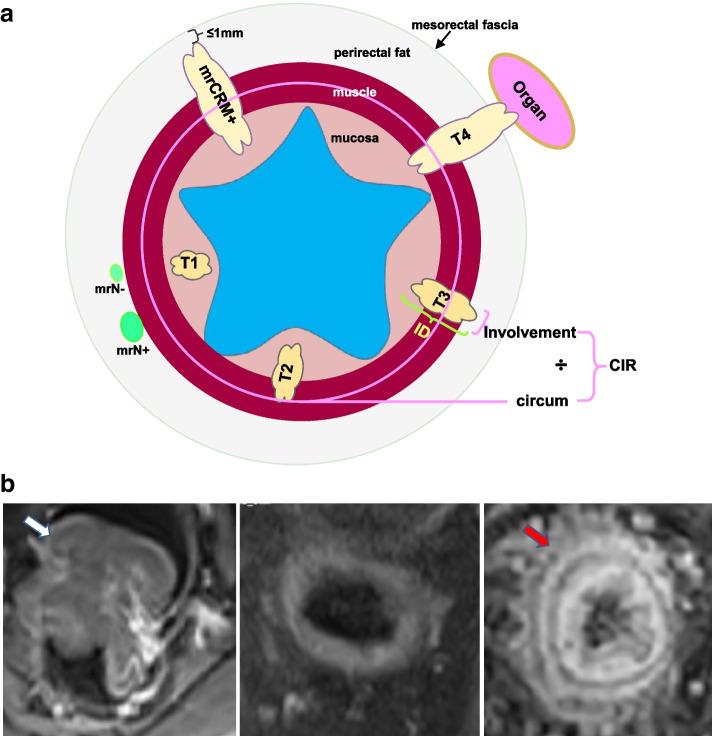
Sketch map of infiltration depth (ID), circum-involvement ratio (CIR), MRI-detected circumferential resection margin (mrCRM), MRI-detected T (mrT) and MRI-detected lymph node (mrN) (a) and enhancement pattern (EP) of rectal cancer (b). Mild/Moderate (left, white arrow) or obvious (right, red arrow) of tumor compared to normal rectal wall enhancement (middle)
